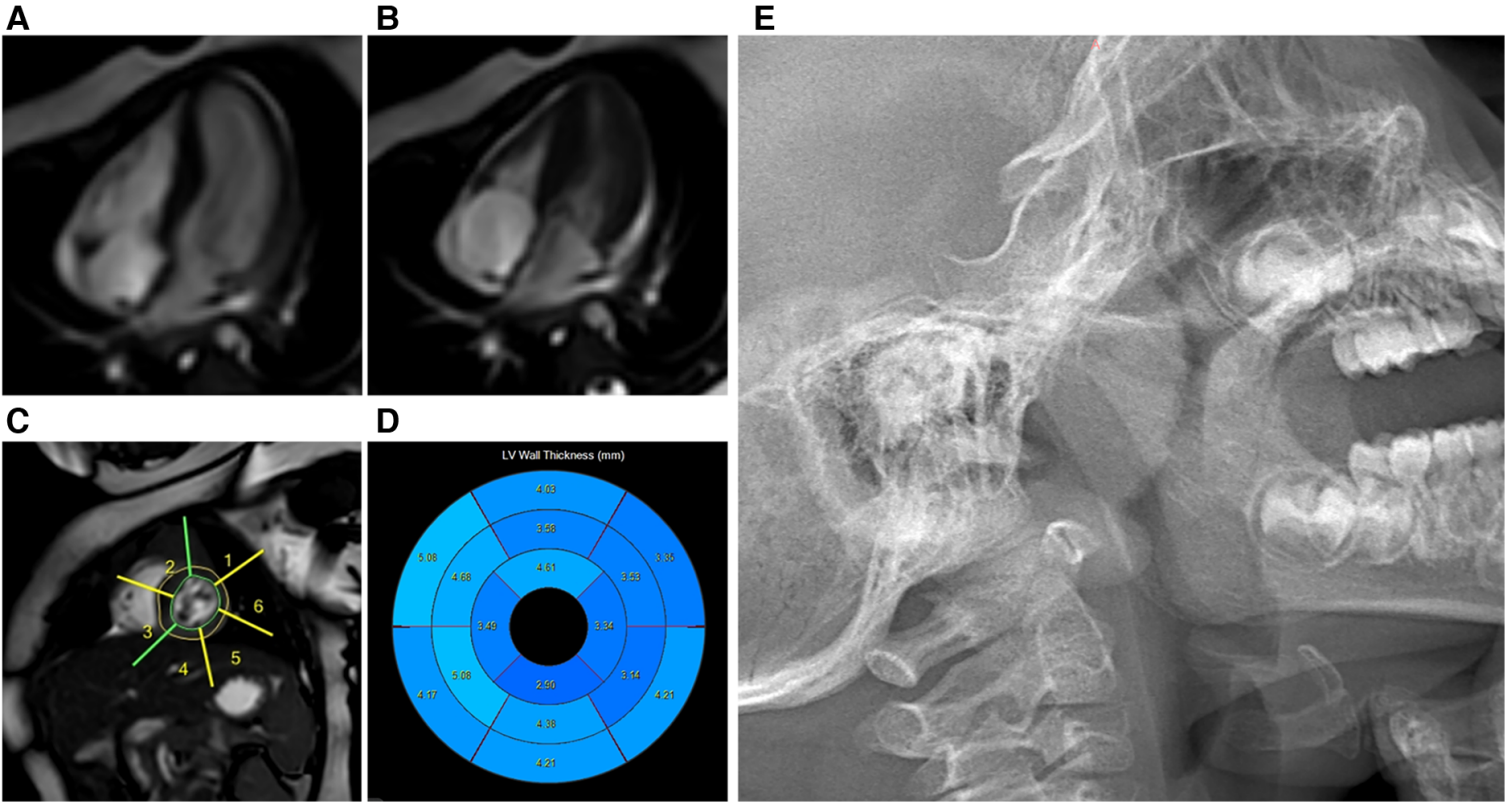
Cardiac MRI: obstructive hypertrophic cardiomyopathy with heterogeneous left ventricular hypertrophy. Cardiac magnetic resonance cine images from mid-ventricular short-axis and four-chamber views at end-diastole (A) and end-systole (B); Cardiac magnetic resonance cine images (C); Bulls-eye plot representing the thickness of the left ventricle (D); Lateral nasopharyngeal x-ray: adenoidal hypertrophy and tonsil hypertrophy (E).
